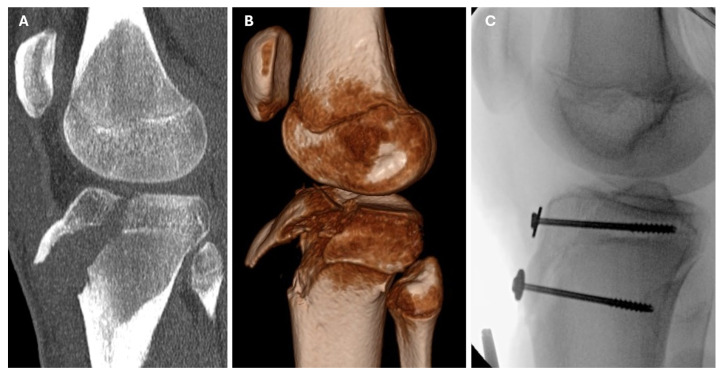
CT, 3D-CT, and intraoperative radiographic views of a type IIIb tibial tuberosity fracture. (A) Sagittal CT scan. (B) A 3D-CT reconstruction view of the fracture. (C) Intraoperative imaging following an open reduction and internal fixation using two cancellous screws and washers.
